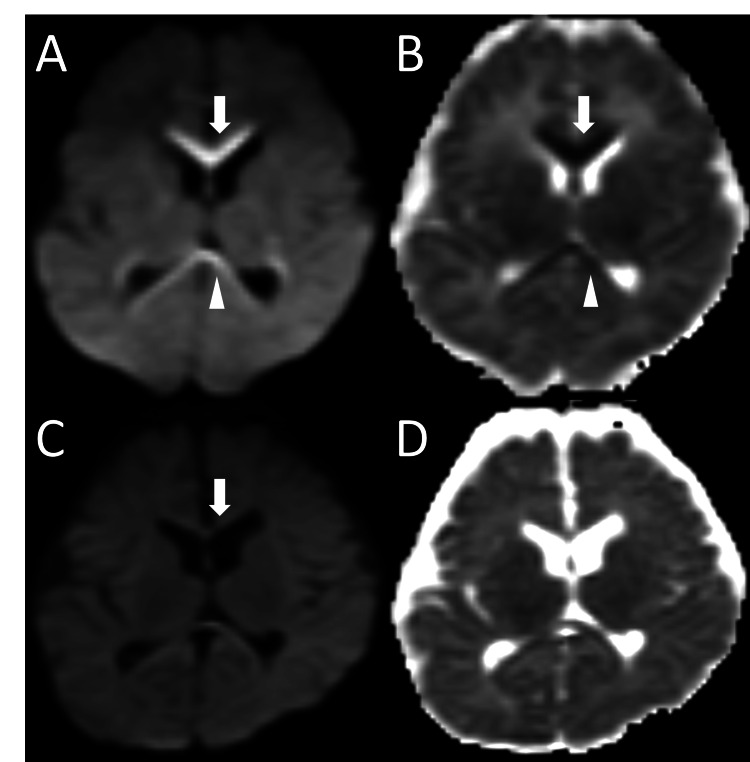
Diffusion restriction in the corpus callosum in congenital myotonic dystrophy Axial diffusion-weighted imaging (DWI) and apparent diffusion coefficient (ADC) maps are shown at the level of the corpus callosum. (A, B) MRI obtained at 32 days of age (hospital day 10) demonstrates high signal intensity in the genu (arrow) and splenium (arrowhead) on DWI (A), with corresponding low signal on the ADC map (B), indicating restricted diffusion. (C, D). A follow-up MRI at four months shows resolution of the previously observed diffusion restriction and mild atrophy of the genu of the corpus callosum (arrow).
